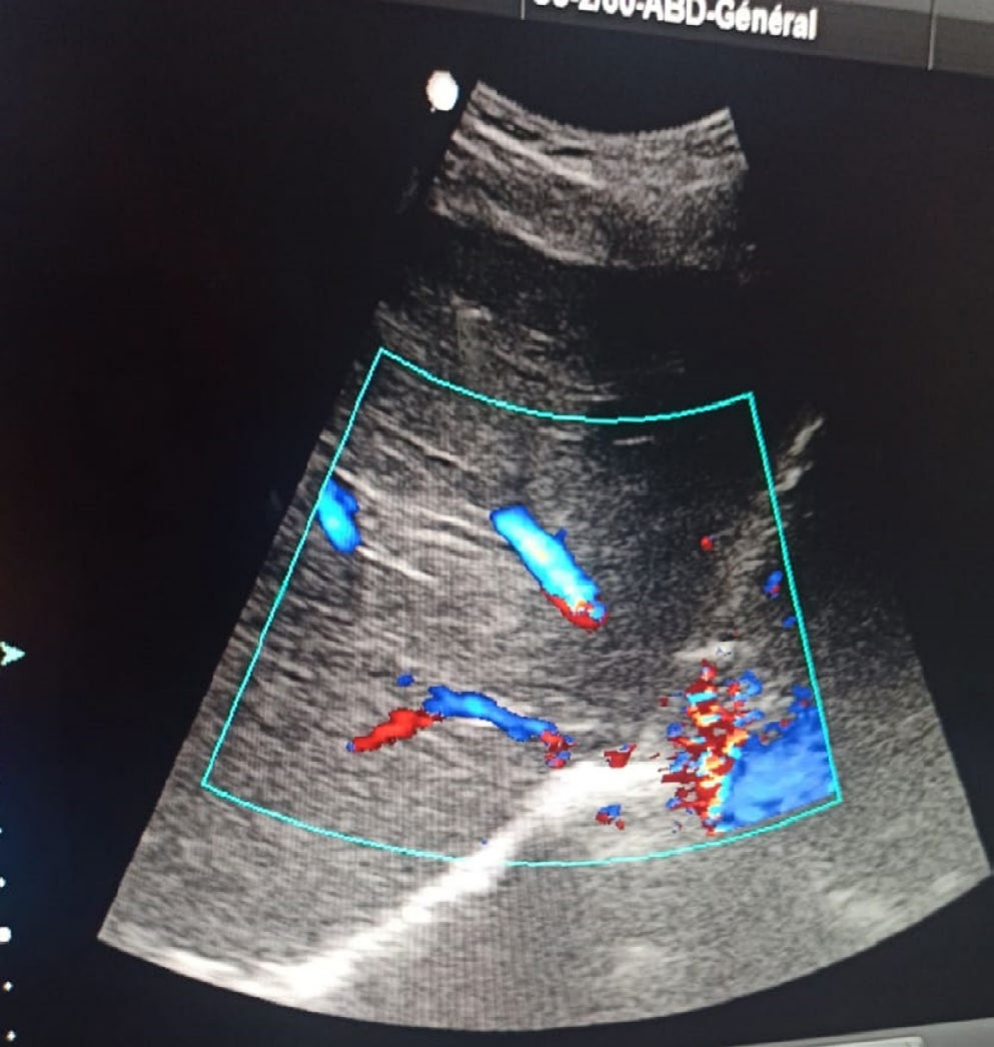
Doppler ultrasound of the liver showing no thrombosis
